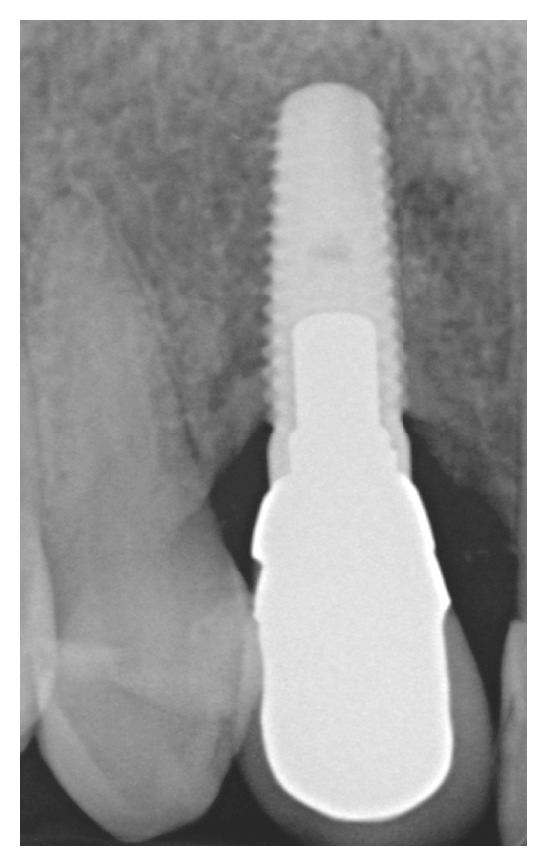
Three-year periapical X-ray. The implant appeared well integrated and the mesial and distal bone levels showed a physiological remodelling.
